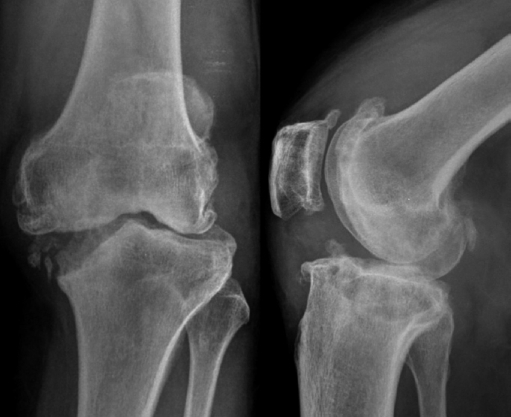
Preoperative radiographs with marked medial tibial bone loss.
